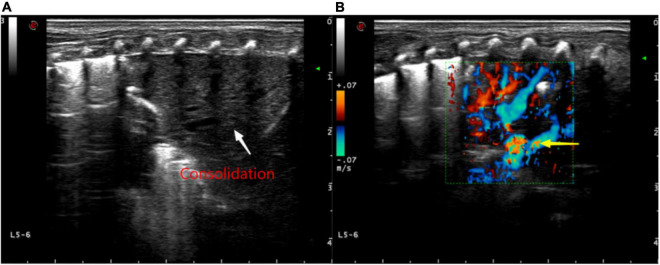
(A) Ultrasound findings of a large consolidation in areas 5–6 of the neonatal left lung. Lung ultrasound shows a large area with the “tissue-like sign” similar to the echo of the liver, spleen, and other tissues (white arrow) and the disappearance of the pleural line and A-line. (B) Color Doppler ultrasound showing blood flow with “dendritic” distribution in the area of consolidation (yellow arrow).
